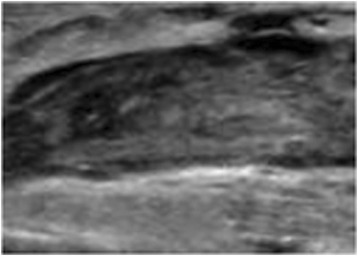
Ultrasound image of thrombosis of right common femoral vein
